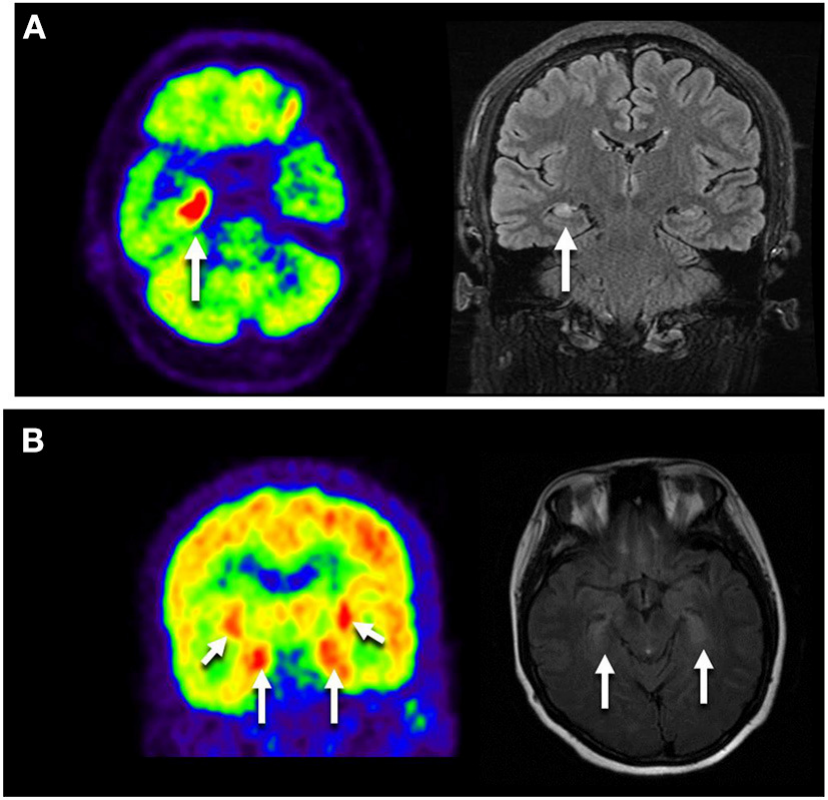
Neuroimages of the GAD65 antibody associated neurological disorders. (A) Images of NO.9 who presented with Ep. Axial FLAIR MRI showed right temporal hyperintensity, and Axial FDG-PET/CT scan showed hypermetabolic right mesiotemporal spot (white arrow). (B) Images of NO.41 who manifested as LE plus ACA. 18F-FDG PET/CT showed increased FDG uptake in the bilateral medial temporal lobes. MRI showed bilateral medial temporal lobe T2 hypertension. LE, limbic encephalitis; Ep, epilepsy; ACA, autoimmune cerebellar ataxia.
